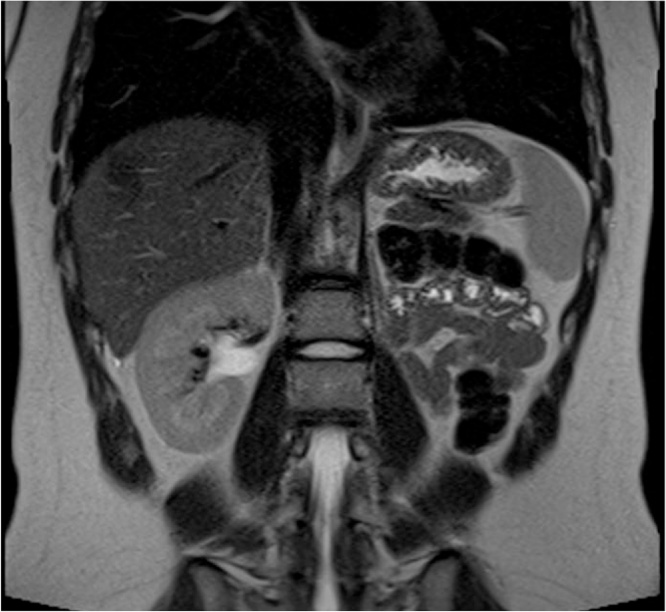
Abdominal MRI, coronal view, showing the absence of the left kidney.
